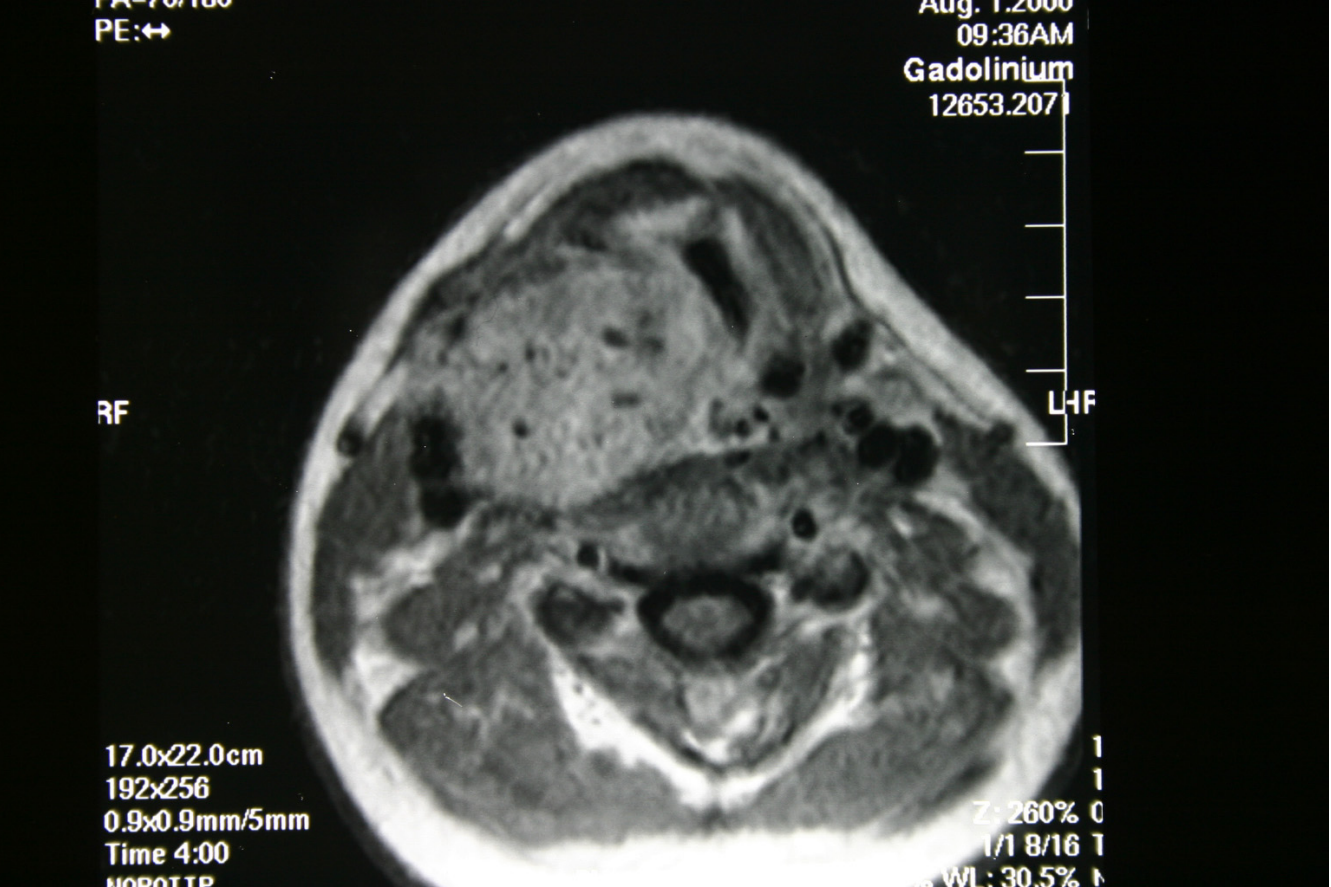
The tumor between the parapharyngeal space and the upper pole of right thyroid lobe was narrowing the airway passage and displacing the carotid artery and the internal jugular vein laterally on MRI.
